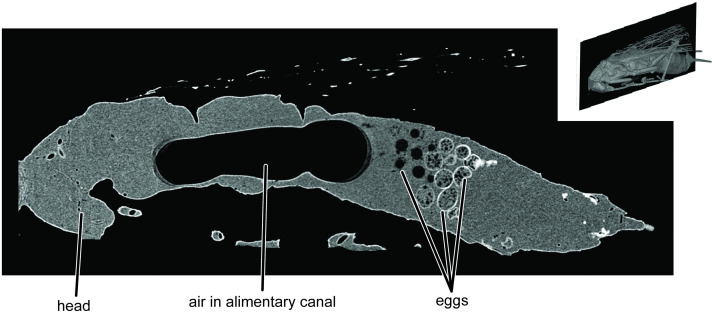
Eggs in abdomen of *Isoperla* sp. (Plecoptera: Perlodidae). Medial longitudinal X-ray section showing position of eggs in abdomen of *Isoperla*, just posterior from air space. (See inset for position of slice.).
